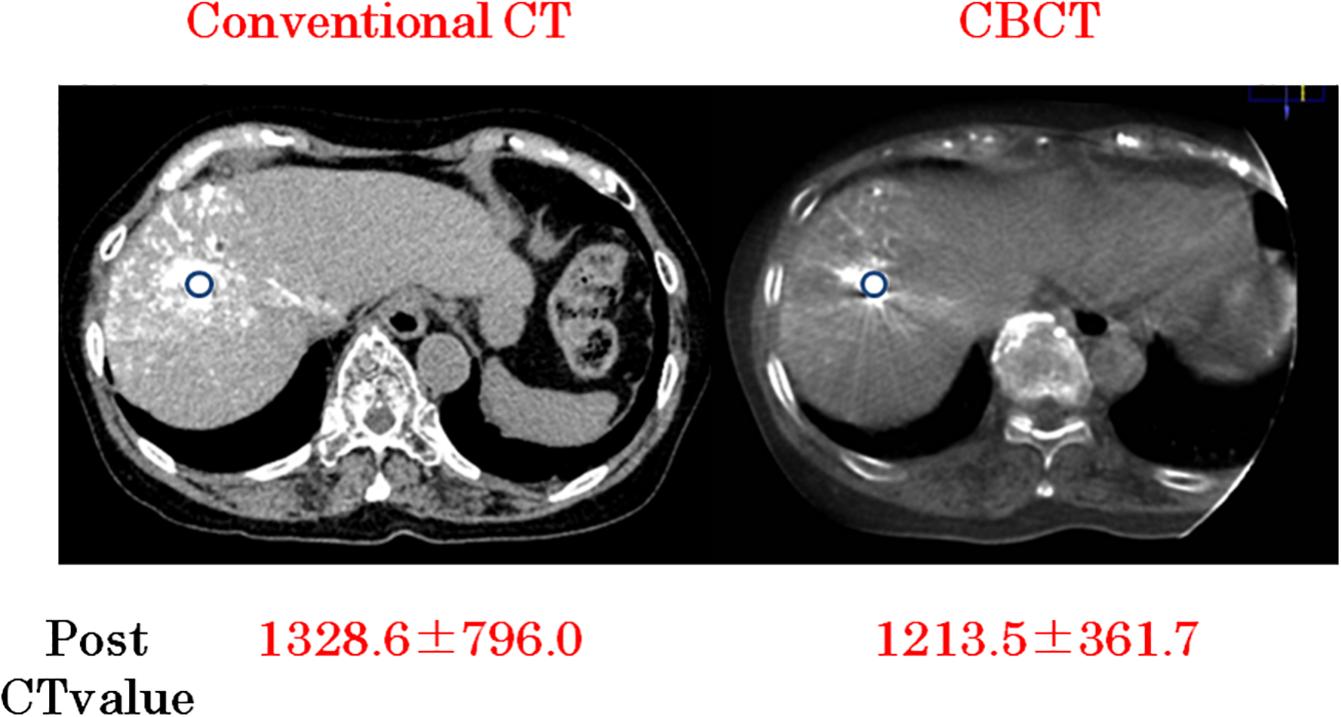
Comparison of ROI on conventional CT (scan parameter; 120kVp, 1366mAs, total scan time; 2.8 seconds, slice thickness; 0.98mm) and CBCT (scan parameter; 119kVp, 165mA, total sweep time; 5.2 seconds, slice thickness; 0.68mm)
